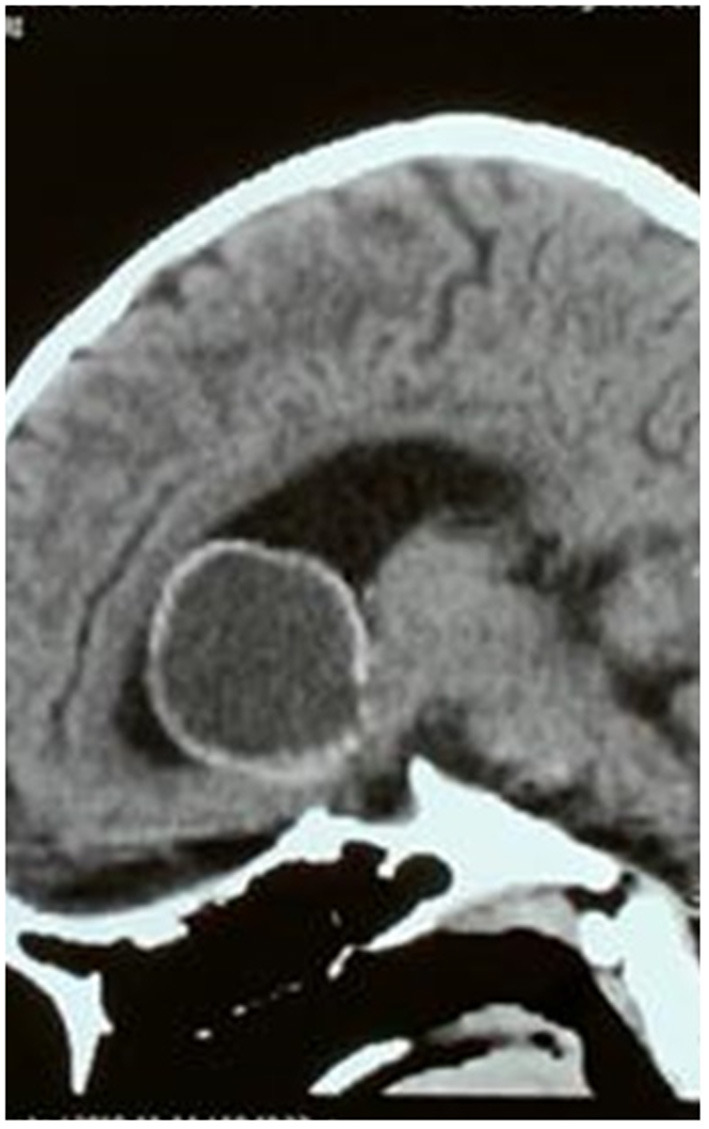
Sagittal section CT scan of a patient with a cystic intraventricular craniopharyngioma showing peripheral calcification, and a bulge in the floor of the third ventricle.
